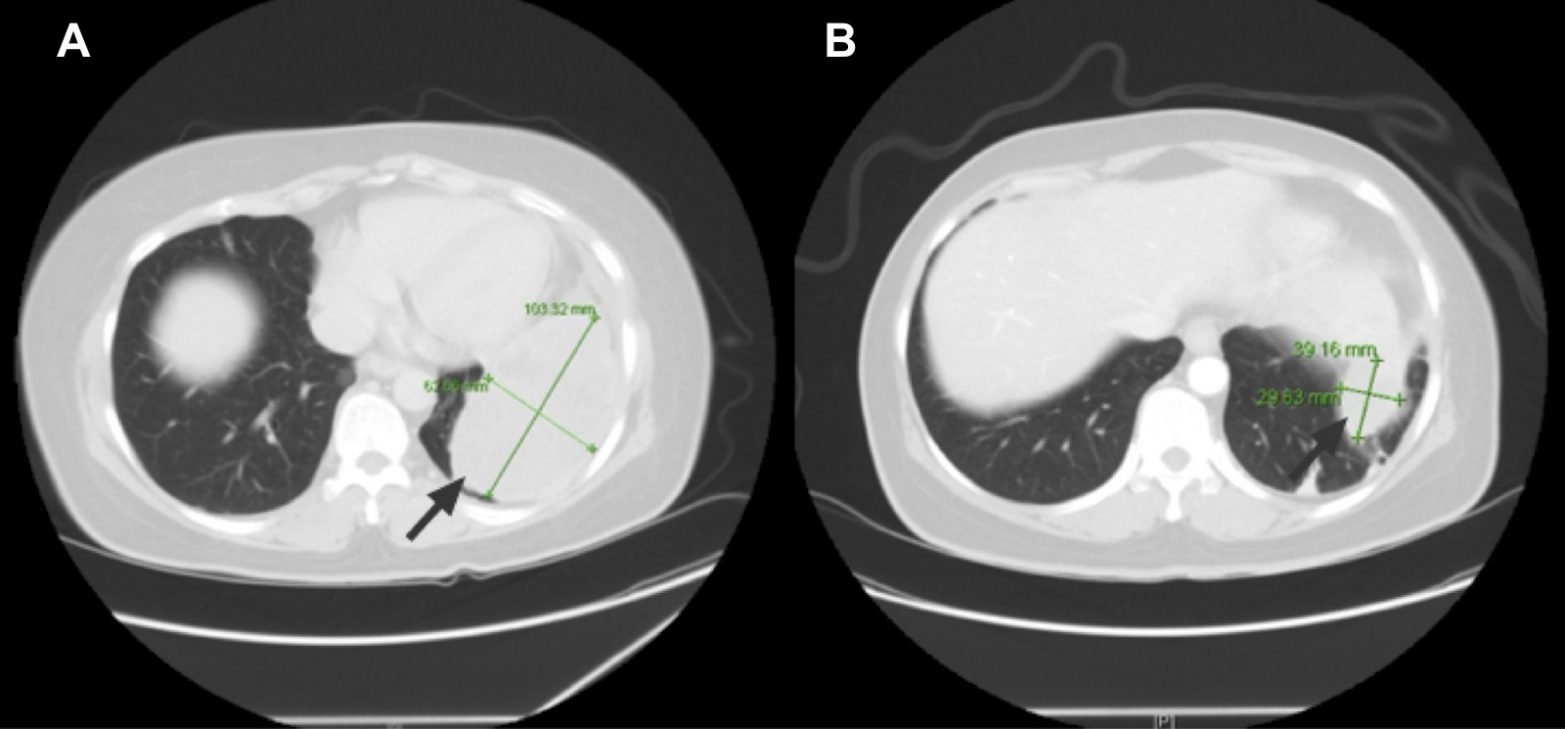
Panel A: CT chest shows a large soft tissue mass at the left lung base (arrow). Panel B: Repeat CT chest post 7 cycles of chemotherapy demonstrates a significant decrease in size of the mass (arrow).
